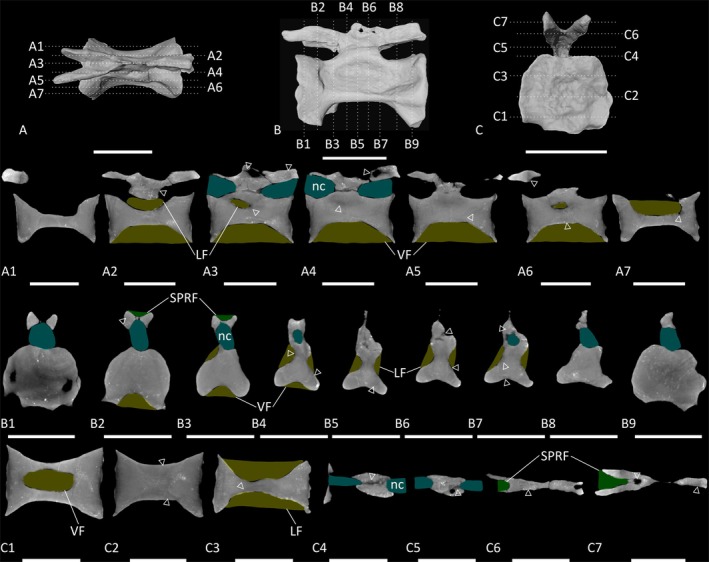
CT scan of *Tornieria africana* posterior caudal vertebra MB.R.2956.19. (A–C) 3D rendering (A) dorsal view, (B) left lateral view, (C) anterior view; (A1–A7) CT scan slices in parasagittal cross‐section, (B1–B9) CT scan slices in transverse cross‐section, (C1–C7) CT scan slices in horizontal cross‐section. LF, lateral fossa; nc, neural canal; SPRF, spinoprezygapophyseal fossa; VF, ventral fossa. Key: Blue highlight, neural canal; green highlight, neural spine fossa; yellow highlight, centrum fossa; white arrow, pneumatic bone texture. Scale bars = 100 mm.
